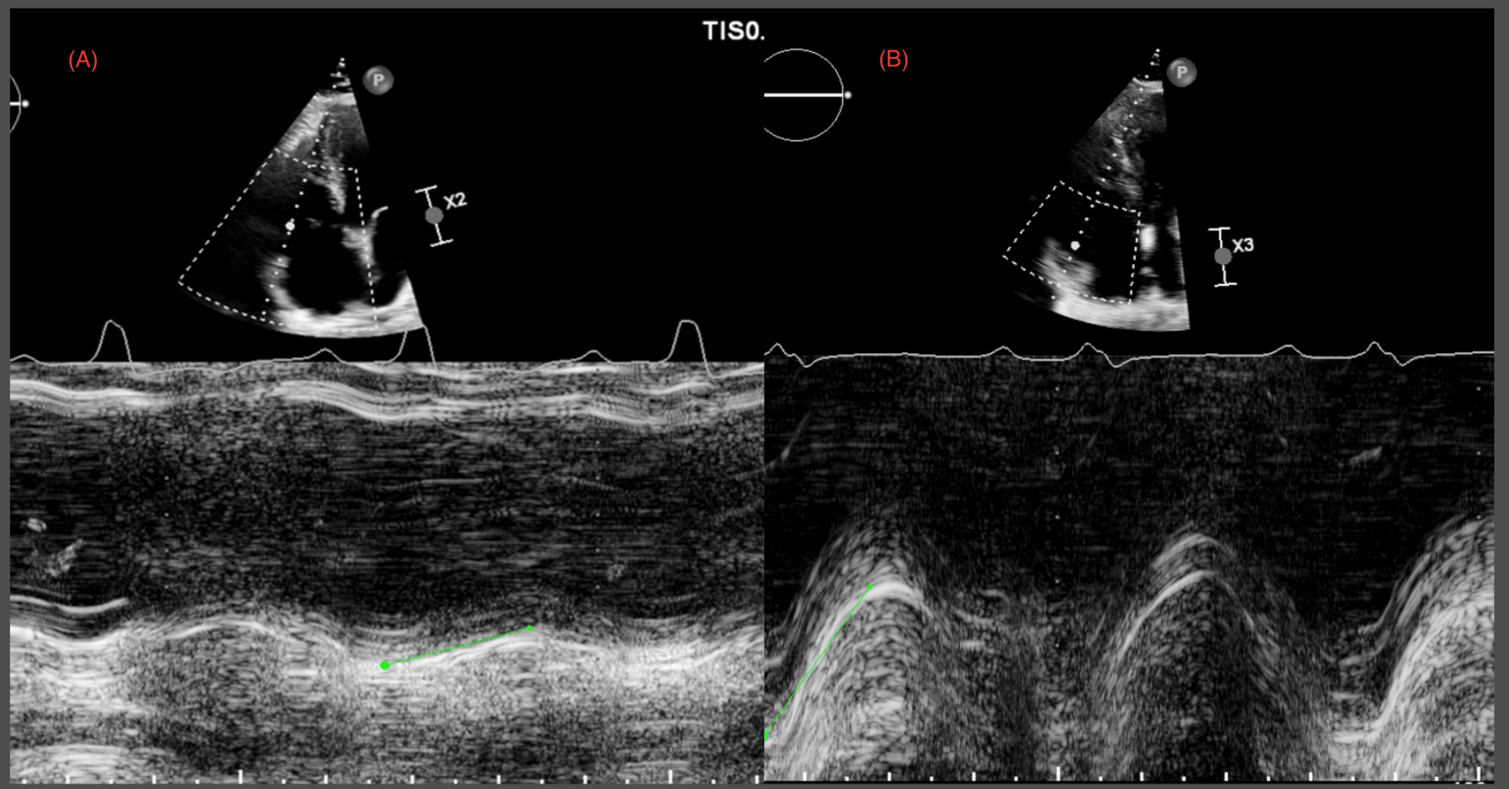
Echocardiography images Before thrombectomy (A) and after thrombectomy (B), revealing normalization of tricuspid annular plane systolic excursion from 1.10 cm to 2.3 cm.
